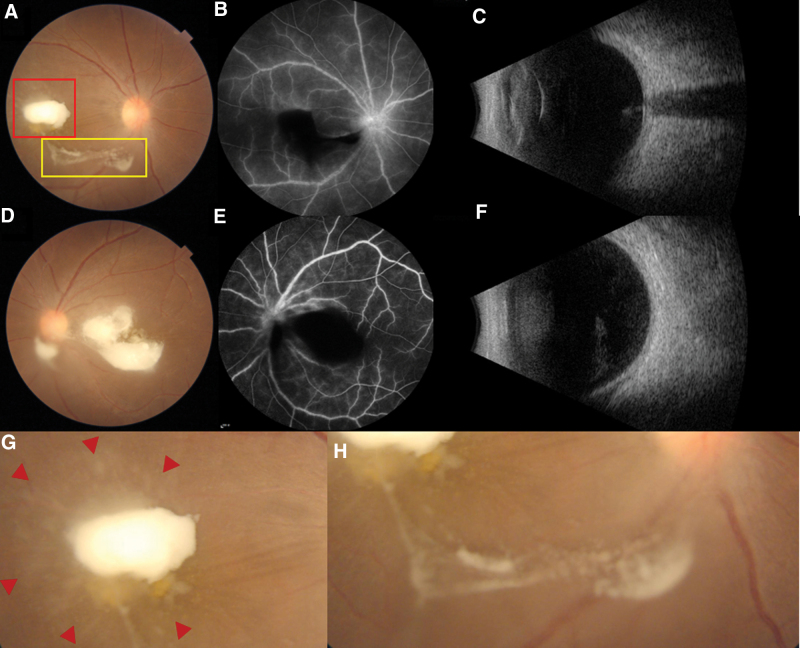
Upon admission fundus examination showed a hazy view of optic disc and posterior retina. There was a whitish mass (Fig. 2A and Fig. 2D) with “string and pearls” (yellow box in Fig. 2A and Fig. 2H) in each eye. The mass in the right eye emit umbrella-like filaments attached to the retina (red box in Fig. 2A and Fig. 2G). FFA showed optic disc and posterior retinal vessels leakage (Fig. 2B and Fig. 2E). Ultrasonic B scan demonstrated dense punctate echoes in the posterior vitreous and flat retina in both eyes (Fig. 2C and Fig. 2F). FFA = fundus fluorescein angiography.
